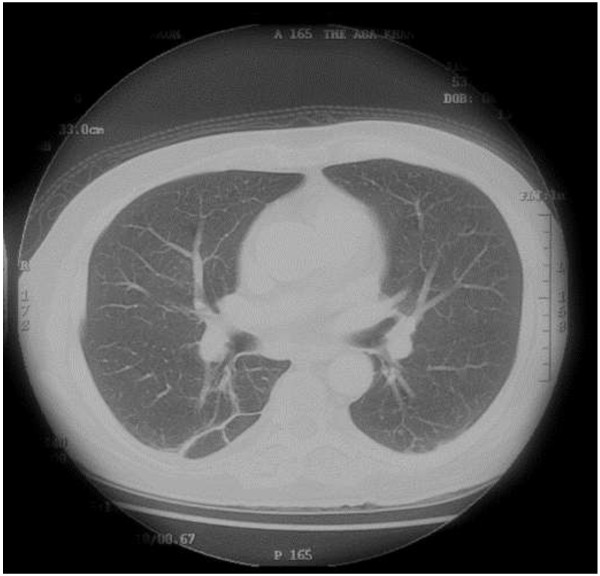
(Case 1) CT Chest after treatment for Nocardia (resolution of cavitatory lesion).
